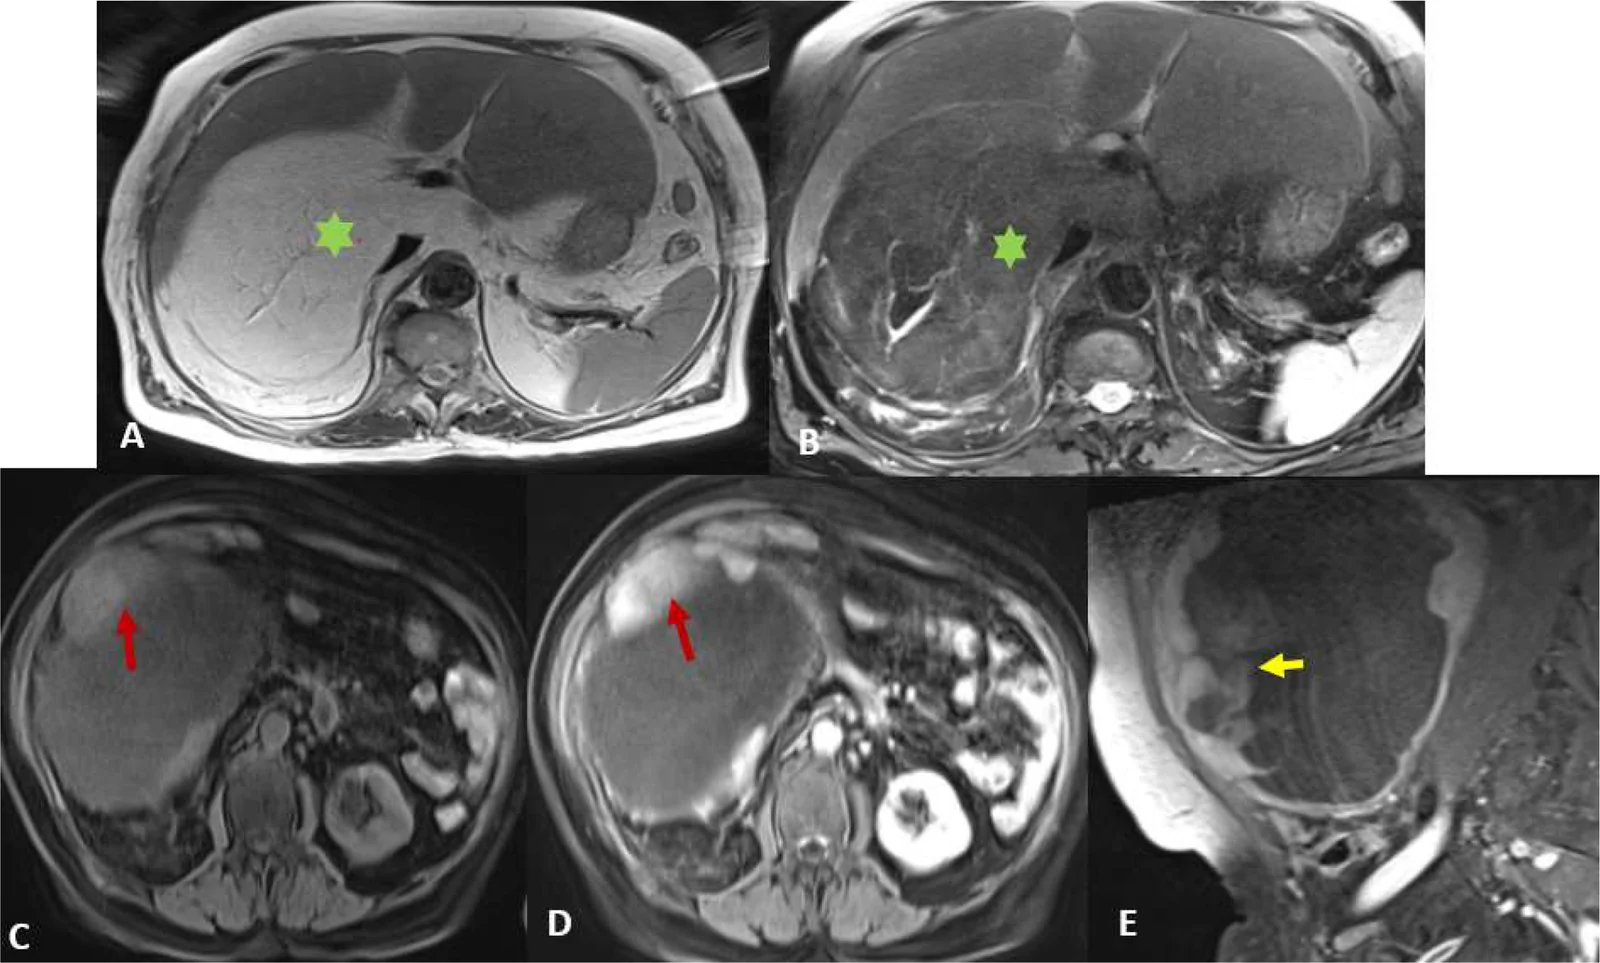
Abdominopelvic MRI, axial T2 weighted sequence without (A) and with fat saturation (B), axial unenhanced T1-weighted sequence (C), axial (D) and sagittal (E) T1 Gadolinium-enhanced sequence showing the fatty portion (green star), thickened septa (yellow arrow), and the enhanced soft tissue portion (red arrow).
